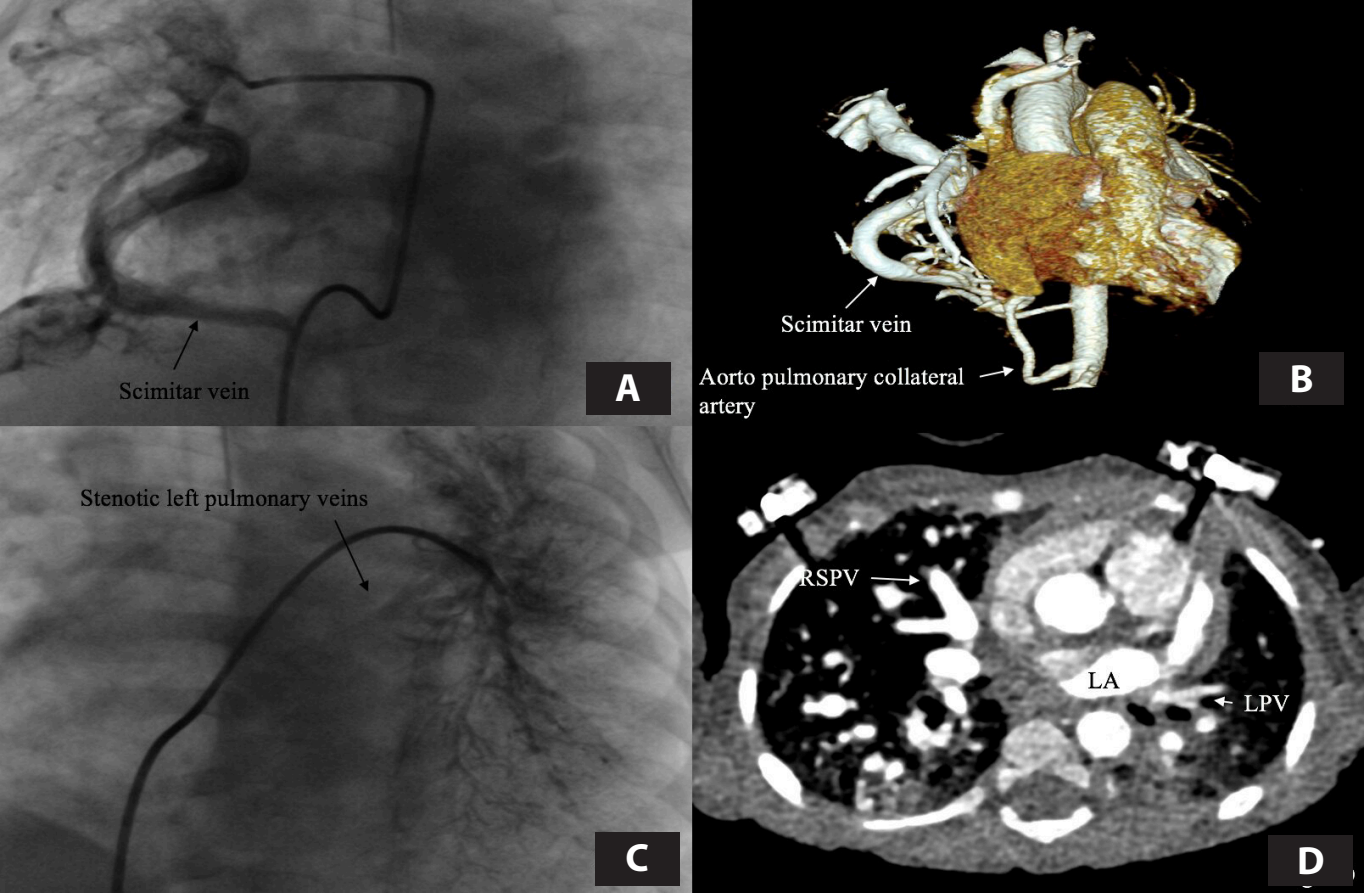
Preoperative computed tomography angiography and cardiac catheterization demonstrating the right lower anomalous pulmonary venous return to the inferior vena cava via the scimitar vein, the aortopulmonary collateral artery from the abdominal aorta to the right lower lung (A,B), and the stenotic left pulmonary veins (C,D). LA=left atrium; LPV=left pulmonary vein; RSPV=right superior pulmonary vein
